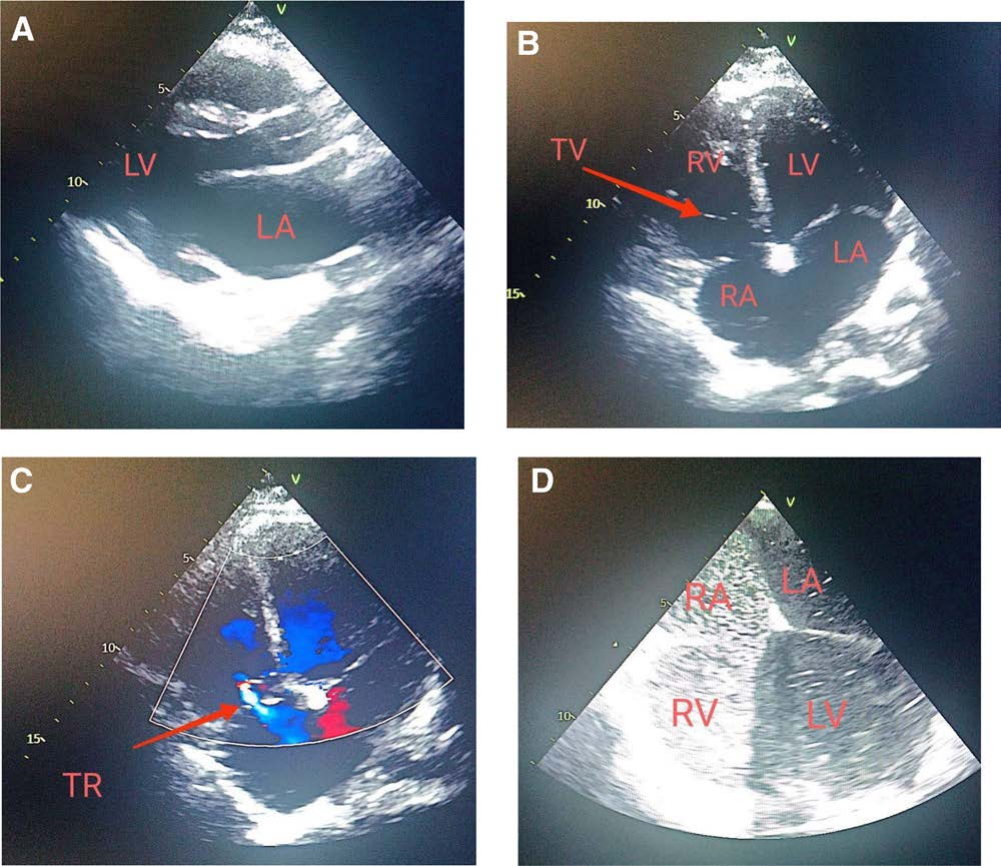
(A) TTE in the parasternal long-axis view showing a mildly dilated LA with preserved left ventricular structure. (B) TTE with apical 4 chambers view showing mildly dilated both atria, and mildly dilated RV. (C) TTE with apical 4 chambers view showing mild TR. (D) TEE showing positive bubble test (during Valsalva maneuver) due to residual shunt post ASD closure. ASD = atrial septal defect, LA = left atrium, LV = left ventricle, RA = right atrium, RV = right ventricle, TEE = transesophageal echocardiogram, TR = tricuspid regurgitation, TTE = transthoracic echocardiogram, TV = tricuspid valve.
